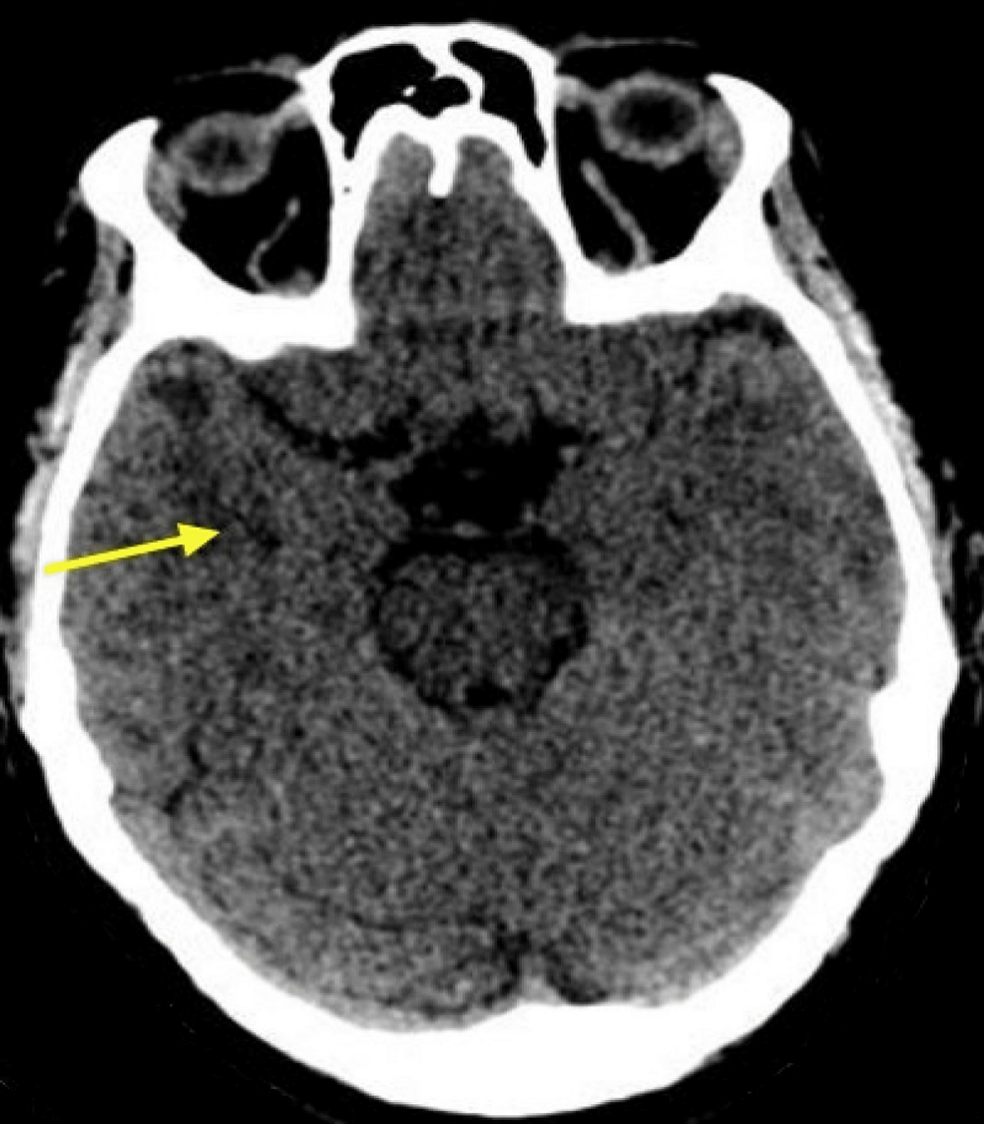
Repeat CT head image after seven days Bilateral anterior temporal lobe infarcts with extensive periventricular and deep white matter low attenuation change throughout the cerebrum with a right-side predominance demonstrated and is more evident than on the previous CT head (yellow arrow).
